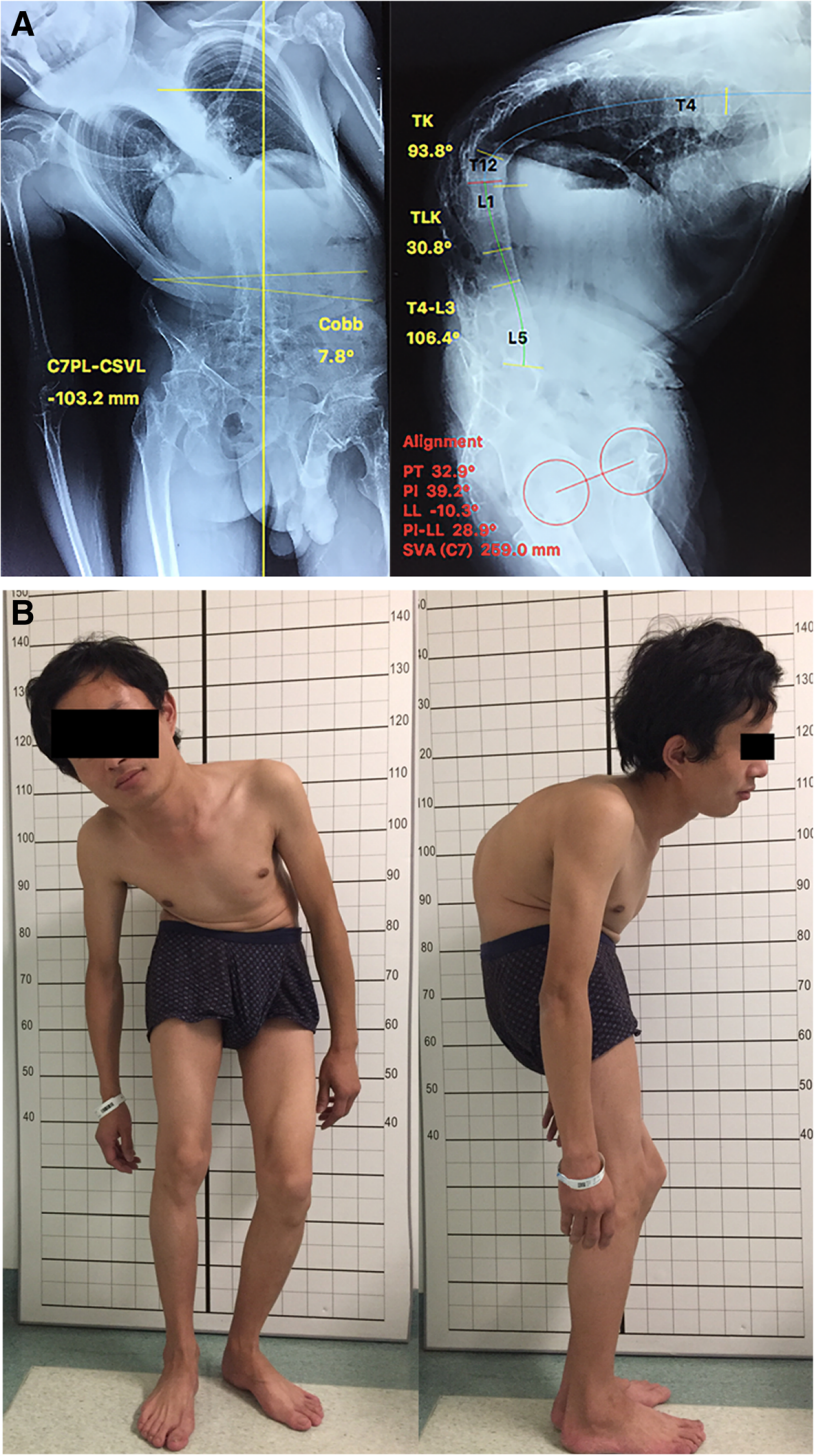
a Preoperative X-ray. TK, PT, PI, and SVA were 93.8°, 32.9°, 39.2°, and 259.0 mm, respectively. The total kyphosis of the spine (T4–L3) was 106.4°. b Preoperative clinical photographs showing the patient with thoracolumbar kyphoscoliosis. His chin-brow vertical angle was 21°
